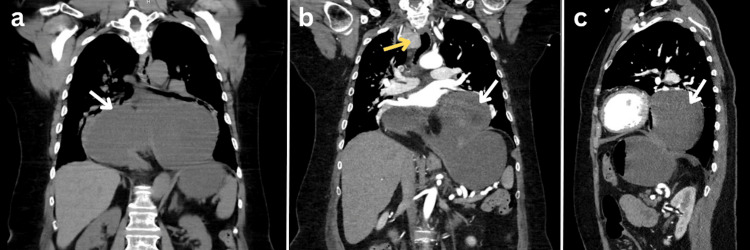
CT of chest, abdomen, and pelvis with and without contrast; (a and b) coronal view, (c) sagittal view Gastric obstruction secondary to a large hiatal hernia with a superimposed organo-axial gastric volvulus (white arrows) and enlarged thyroid with a mild right to left tracheal deviation (yellow arrow).
